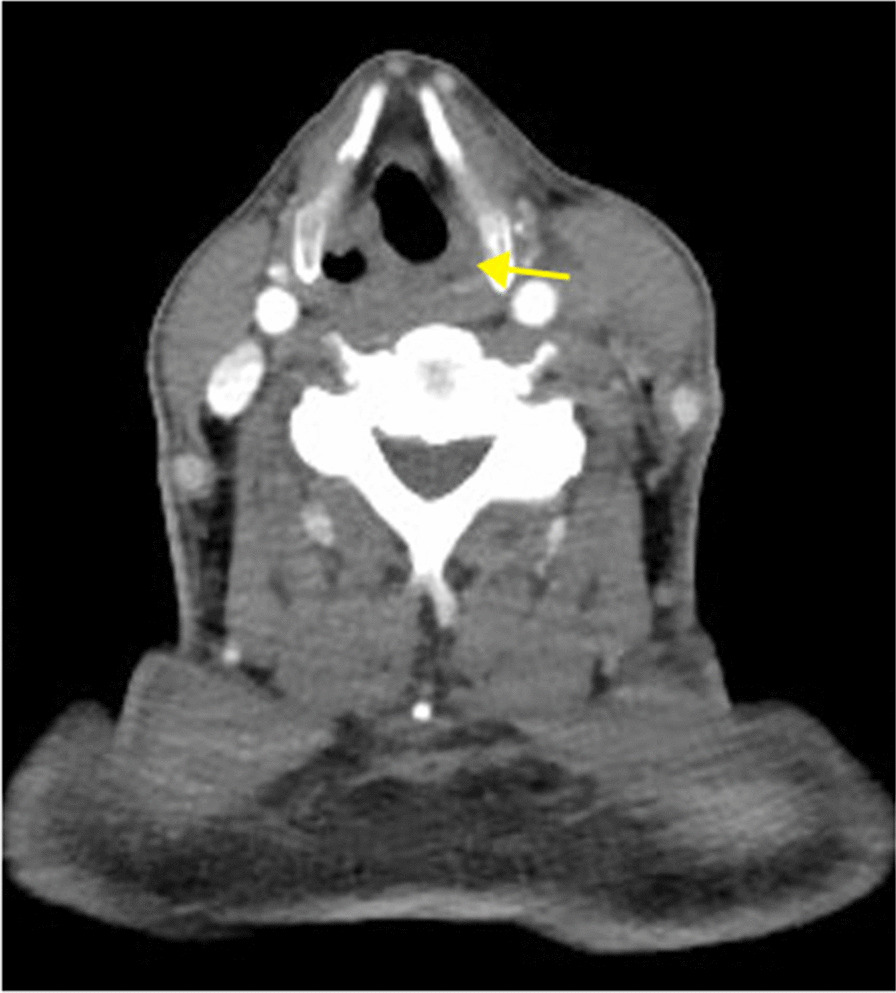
Coronal plane of computed tomography showed disappearance of left pyriform sinus surrounding by abnormal enhancement (yellow arrow)
